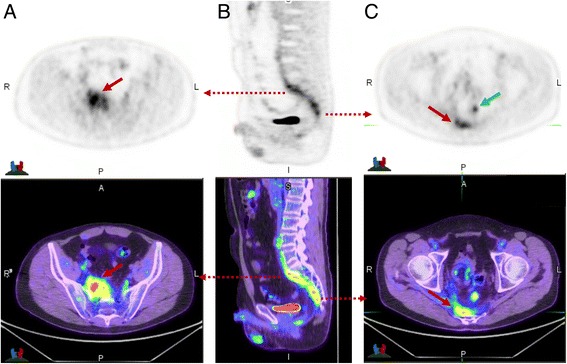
This patient had suspicion of pelvic recurrence in the setting of prior surgical excision for rectal carcinoma. There was intense uptake in the known pre-sacral soft tissue thickening (a) and (c) (red arrow) with SUVmax of 11. The linear morphology on the coronal image (b) suggested this was more likely inflammatory than malignant. A separate linear tract of metabolic activity was also seen (green arrow) extending from the pre-sacral abnormality to the peri-anal region (not shown). All abnormalities resolved following antiobiotic therapy confirming inflammatory aetiology
